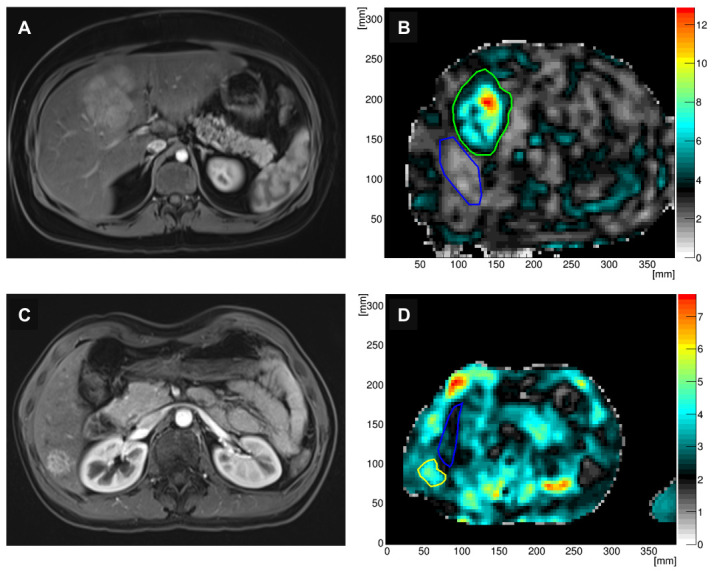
Representative magnetic resonance imaging and elastography findings in benign focal liver lesions. (A) Axial contrast-enhanced T1-weighted MR image in the hepatobiliary phase demonstrating a focal nodular hyperplasia. (B) Corresponding three-dimensional magnetic resonance elastography stiffness map showing elevated stiffness within the lesion (green ROI) relative to surrounding liver parenchyma (blue ROI). (C) Axial contrast-enhanced T1-weighted MR image of a hepatic adenoma. (D) Corresponding stiffness map with regions of interest placed within the lesion (green ROI) and adjacent liver tissue (blue ROI). Note the matched field of view and orientation between anatomical and parametric images. ROI, region of interest.
